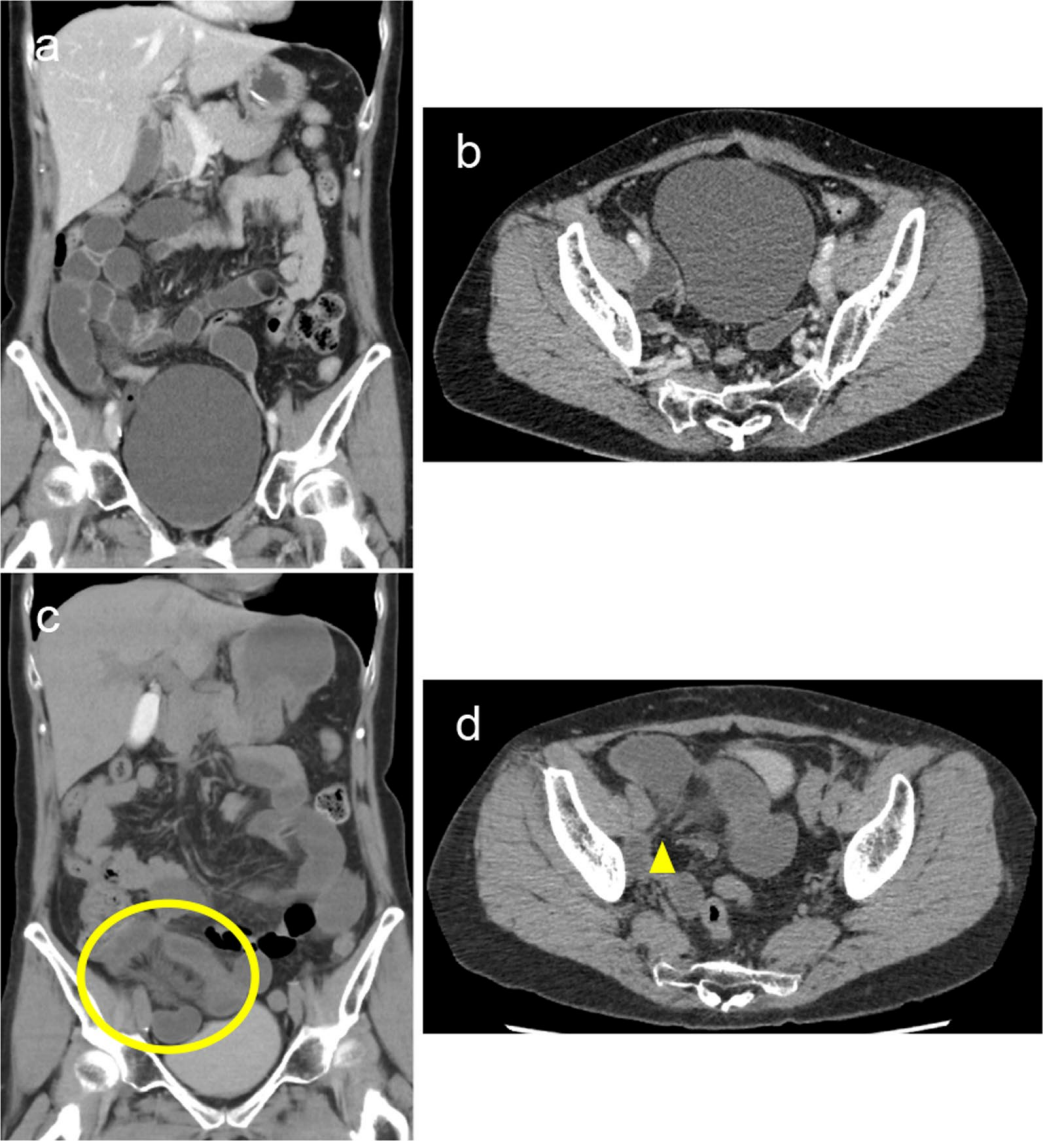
Abdominal CT scan images of the case 1 patient taken at her first visit (a, b) and 6 h after hospitalization (c, d). a and b Coronal (a) and axial (b) enhanced CT scan images at her first visit showed dilated small intestine without a closed loop. c and d Coronal (c) and axial (d) plain CT scan images 6 h after the hospitalization exhibited edematous mesentery (yellow circle) and a closed loop, the origin of which was in the right pelvic wall (yellow arrowhead)
